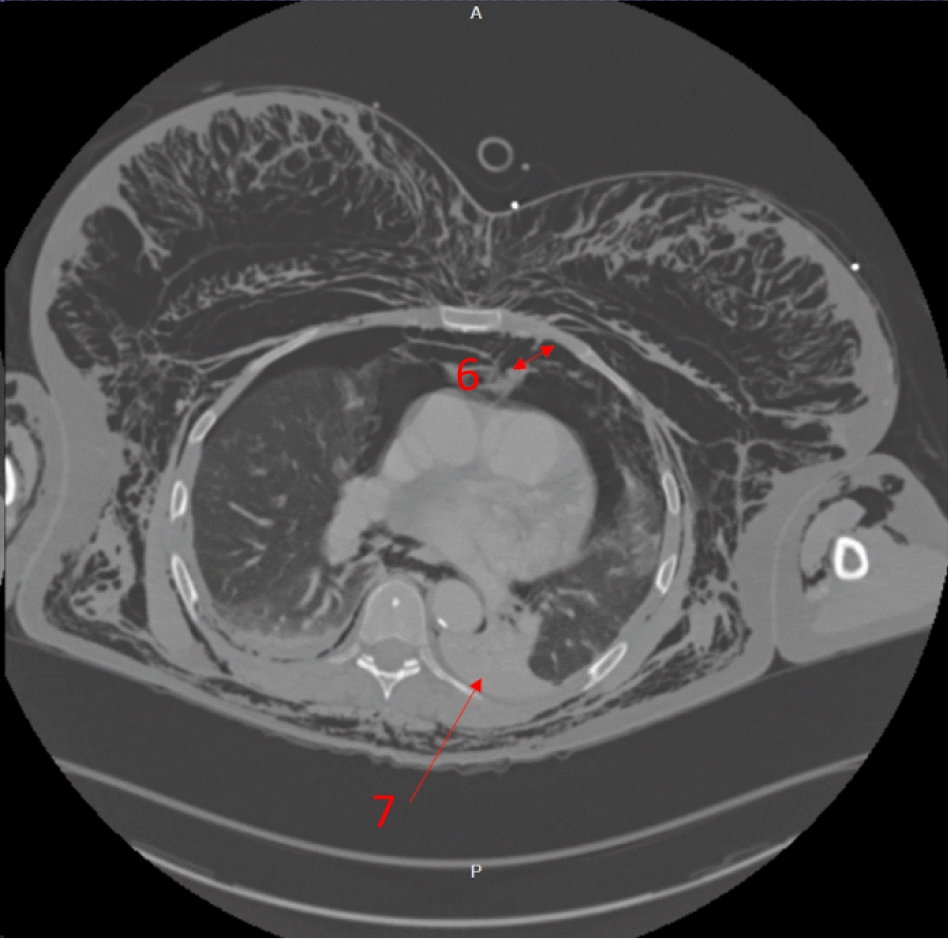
Axial computed tomography showing the left-sided moderate-volume pneumothorax (arrow 6) and the left-sided lower-lobe laceration and collapse (arrow 7)
